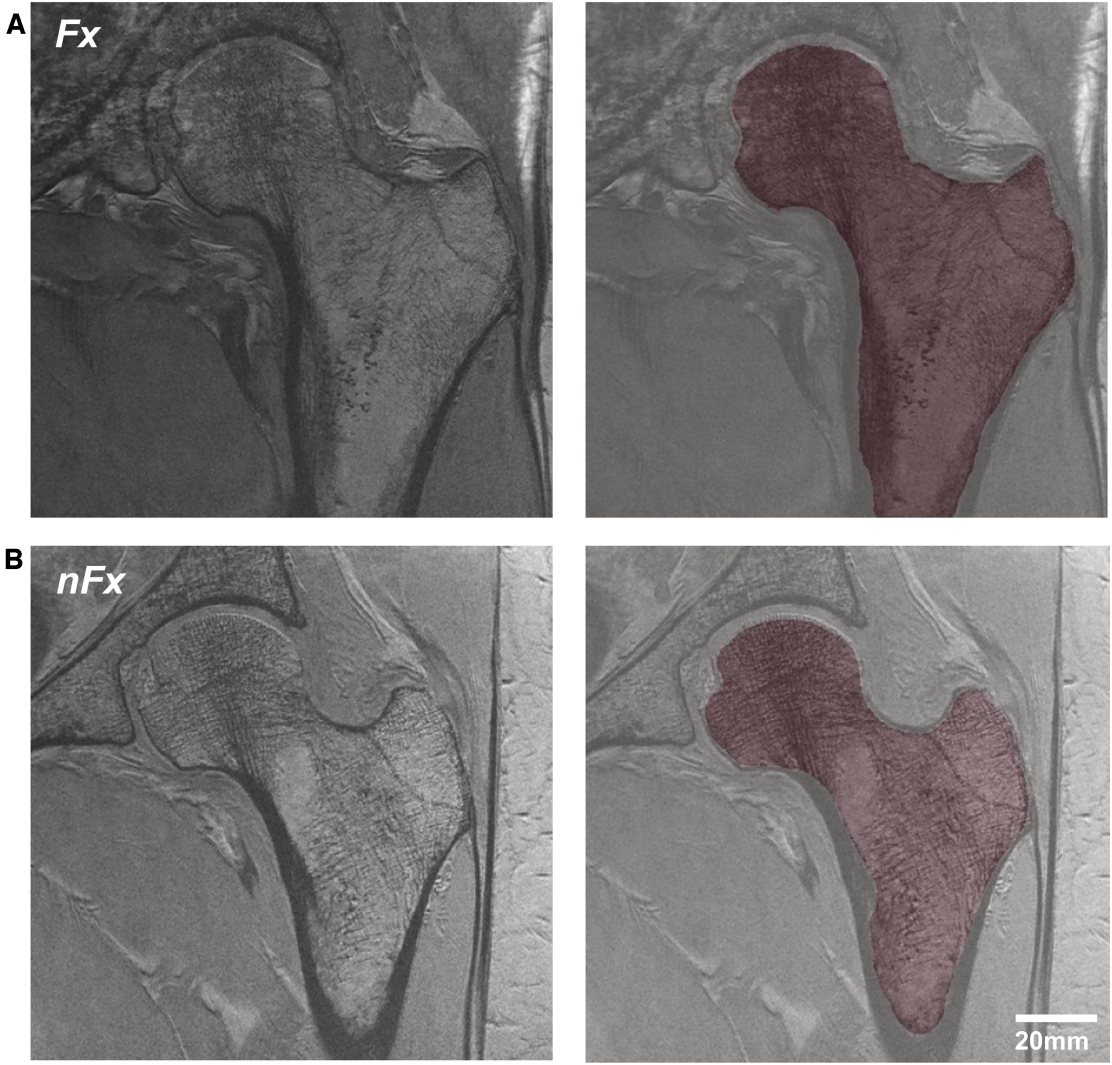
MRI acquisition of the proximal femur and segmented trabecular region in osteoporosis patients, differentiated by (A) history of previous fracture and (B) no fracture history.
